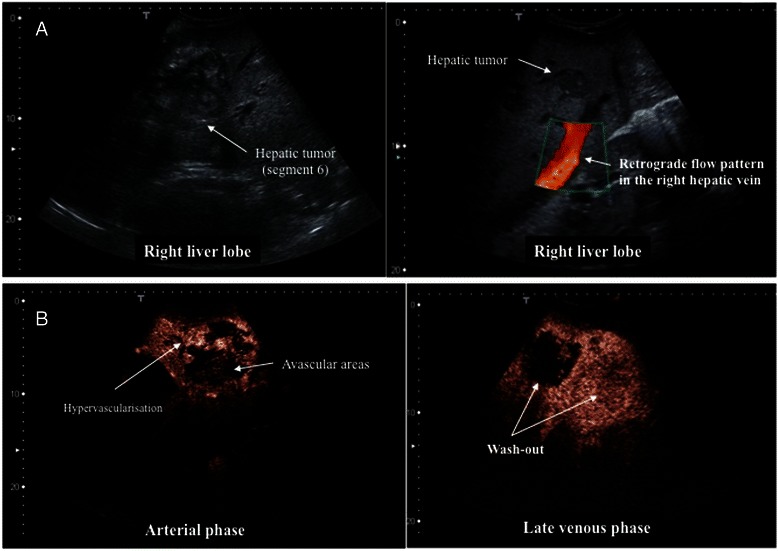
a B-mode ultrasonography: The hepatic tumors were polycyclic, isoechoic and inhomogeneous. Doppler sonography showed a compression in the IVC hepatic segment by the liver tumors with consecutive development of partial Budd Chiari syndrome diagnosed by the retrograde flow pattern in the right hepatic vein and the presence of subcapsular venous collaterals. b Contrast enhanced ultrasonography (CEUS): CEUS was performed using a bolus injection of 1.5 mL SonoVue® (Bracco SpA, Milan, Italy). The largest lesion showed a large, avascular center with hypervascular margin in the arterial phase. In the late venous phase parts of the center and the margin showed wash-out
